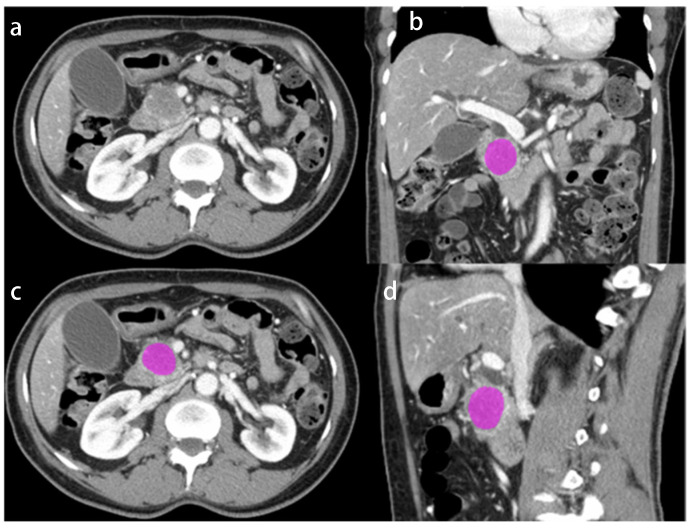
Axial CT image (a) and triplanar CT images with segmentation (displayed in pink; b, c, d) in a representative 62-year-old woman with PDAC in the pancreatic head.
